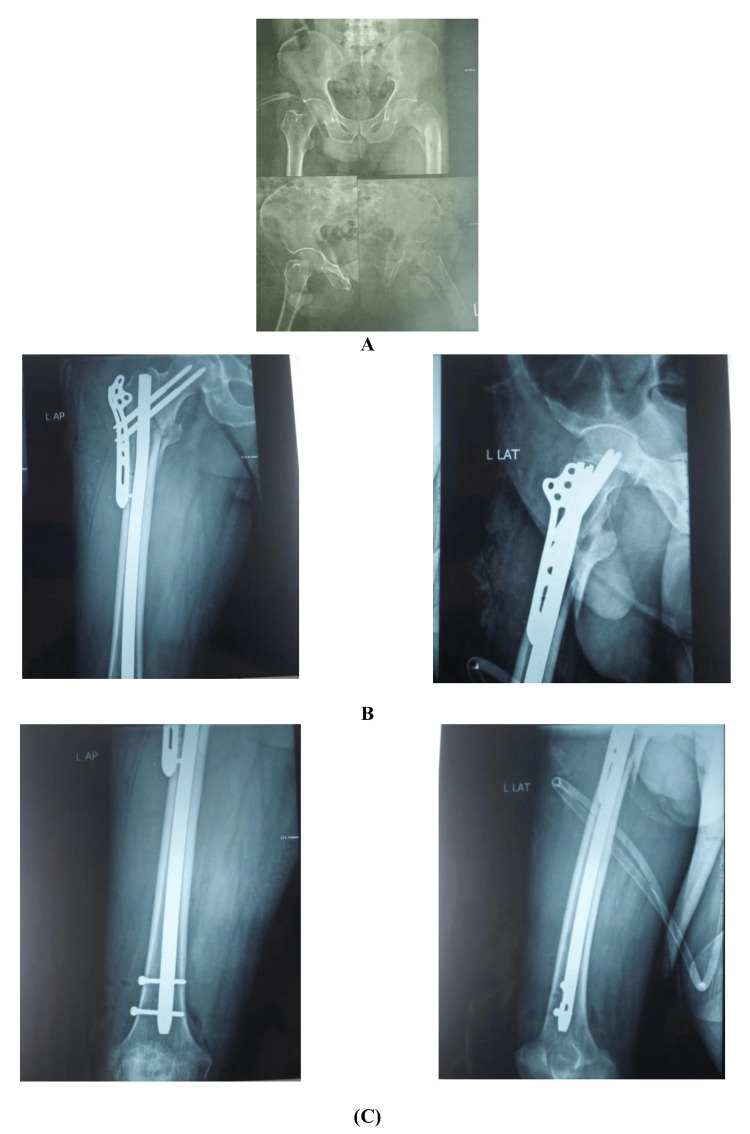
Usage of long nail (A) Preoperative X-rays show an unstable inter-trochanteric femur fracture. (B) Postoperative anteroposterior X-ray shows a nicely reduced fracture. (C) Anteroposterior postoperative X-ray shows the length of the nail, and the lateral postoperative X-ray shows the length of the nail.
